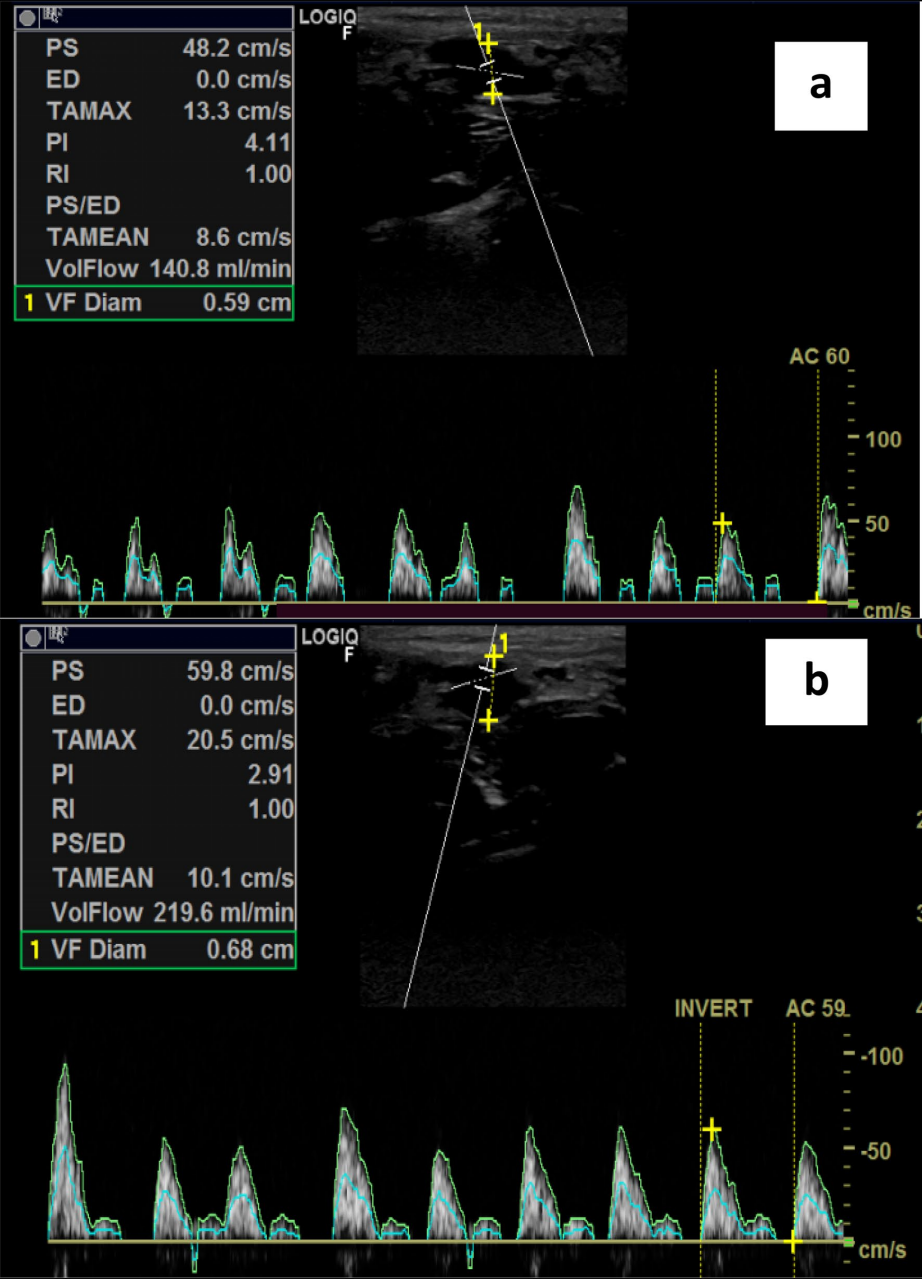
Example of percentage of flow mediated dilatation calculation. a B mode pre-hyperemic sonogram of the arm taken 5 cm proximal to the cubital fossa showing the lumen-intima interface diameter measured as predeflation diameter. b B mode post-hyperemic sonogram of the arm taken 5 cm proximal to the cubital fossa showing the lumen–intima interface diameter measured as post-deflation diameter
